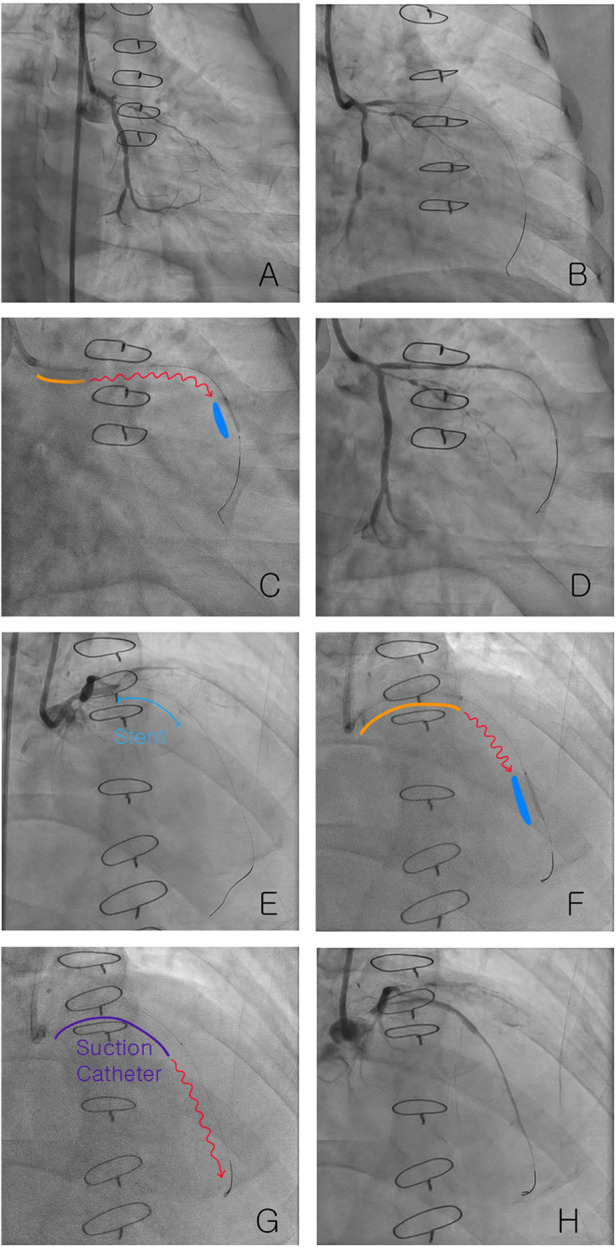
Percutaneous coronary intervention. (A–D) is from caudal view, while (E–H) is from cranial view. (A) Initial angiography showing total occlusion of the proximal LAD. (B) Angiography after multiple balloon predilations and thrombus aspiration, showing no improvement; immediately after intracoronary nicorandil (2 mg) injection, severe chest pain occurred, followed by ventricular fibrillation requiring defibrillation and initiation of VA-ECMO. (C) First Marinade technique session using a GuideLiner extension catheter positioned at the onset of no-reflow, distal occlusion with a 2.5-mm balloon, and intracoronary alteplase (5 mg) with a 5-minute dwell time. (D) Improved angiographic flow after two additional Marinade technique sessions using alteplase (5 mg each). (E) Angiography after intravascular ultrasound-guided deployment of a 2.75 × 33 mm drug-eluting stent, demonstrating recurrent no-reflow. (F) Additional Marinade technique sessions with alteplase (5 mg and 3 mg). (G) Persistent distal no-reflow treated with selective intracoronary alteplase (2 mg) via thrombus aspiration catheter. (H) Final angiography showing sluggish but adequate distal coronary flow (TIMI 2–3).
